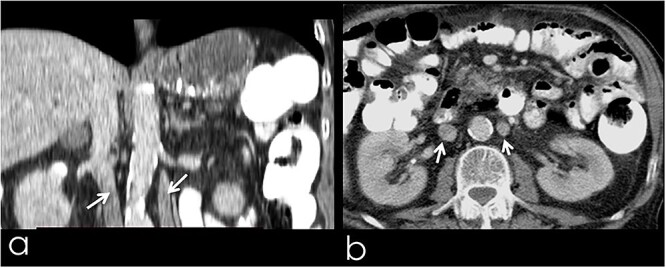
(a) Coronal image from CT with contrast, presenting the duplicated IVC (arrows); (b) transverse image from CT with contrast, presenting the duplicated IVC (arrows).
